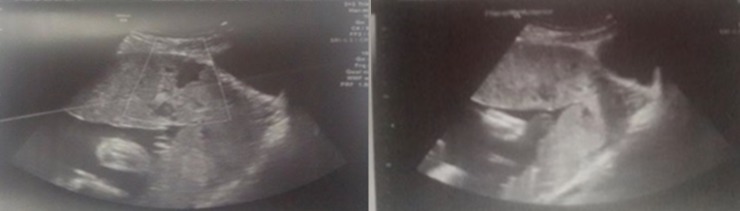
Ultrasound images suggestive of placenta percreta
